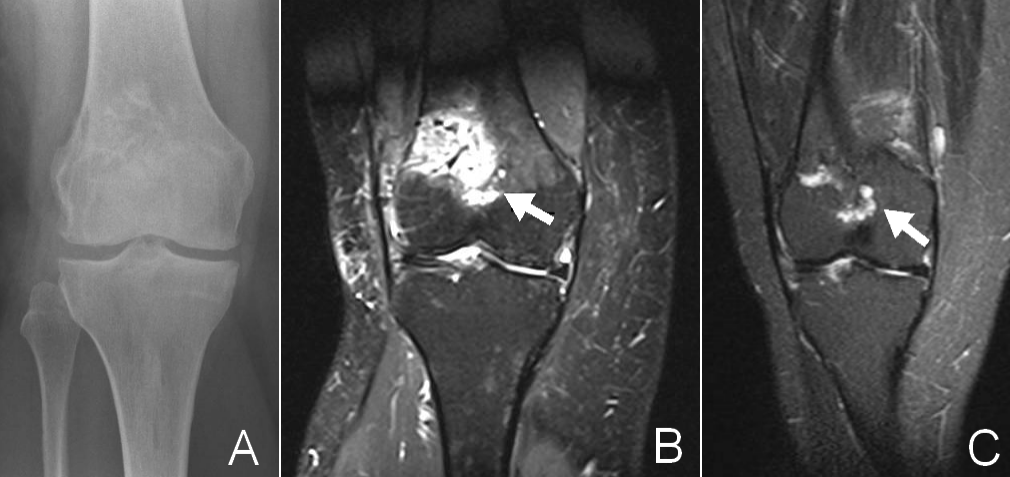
Residue. Series of images of a female patient after a CAS procedure for CHS1 in the right distal femur. A: the post-operative radiograph (Follow-up: 0 days). The defect is reconstructed with a bonechips (bonegraft). No residue visible. B: shows the baseline MRI scan of the same patient (follow-up 3 months). The depicted TIRM sequence shows a reactive response to the graft, with local edema. However below the reconstruction is another lobular, fluid rich structure: retrospectively suggestive forlocal residue but the radiologist describes it as most likely a postoperative reaction of the bone. Further radiographic follow-up shows no recurrence. C: TIRM sequence of the same patient (follow-up: 25 months) shows a nearly died down reaction to the graft but a comparable reaction in the suspected zone. Biopsy before RFA treatment confirmed CHS-1 tissue.
